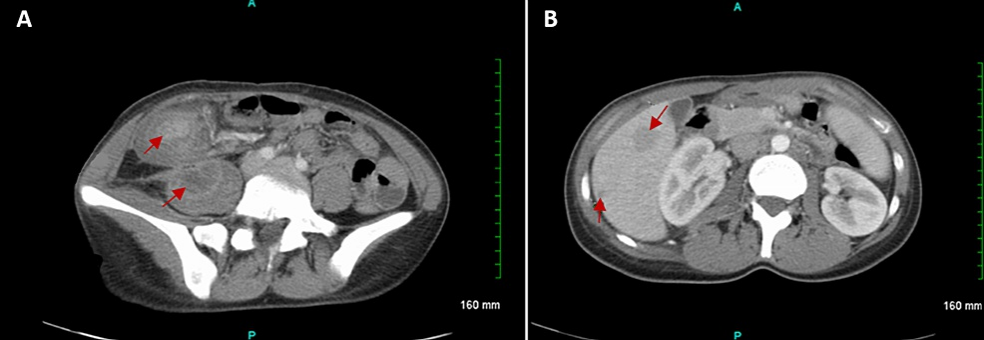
CT scan of the abdomen and pelvis with contrast (A) Abnormal circumferential enhancement edematous changes involving portion of the ascending colon and the cecum with direct involvement of the terminal ileum and the appendix (red arrow). Trace free fluid in the pelvis. Heterogenous enhancement involving the psoas muscle measuring 4.2 cm x 2.8 cm suggestive of abscess (red arrow).
